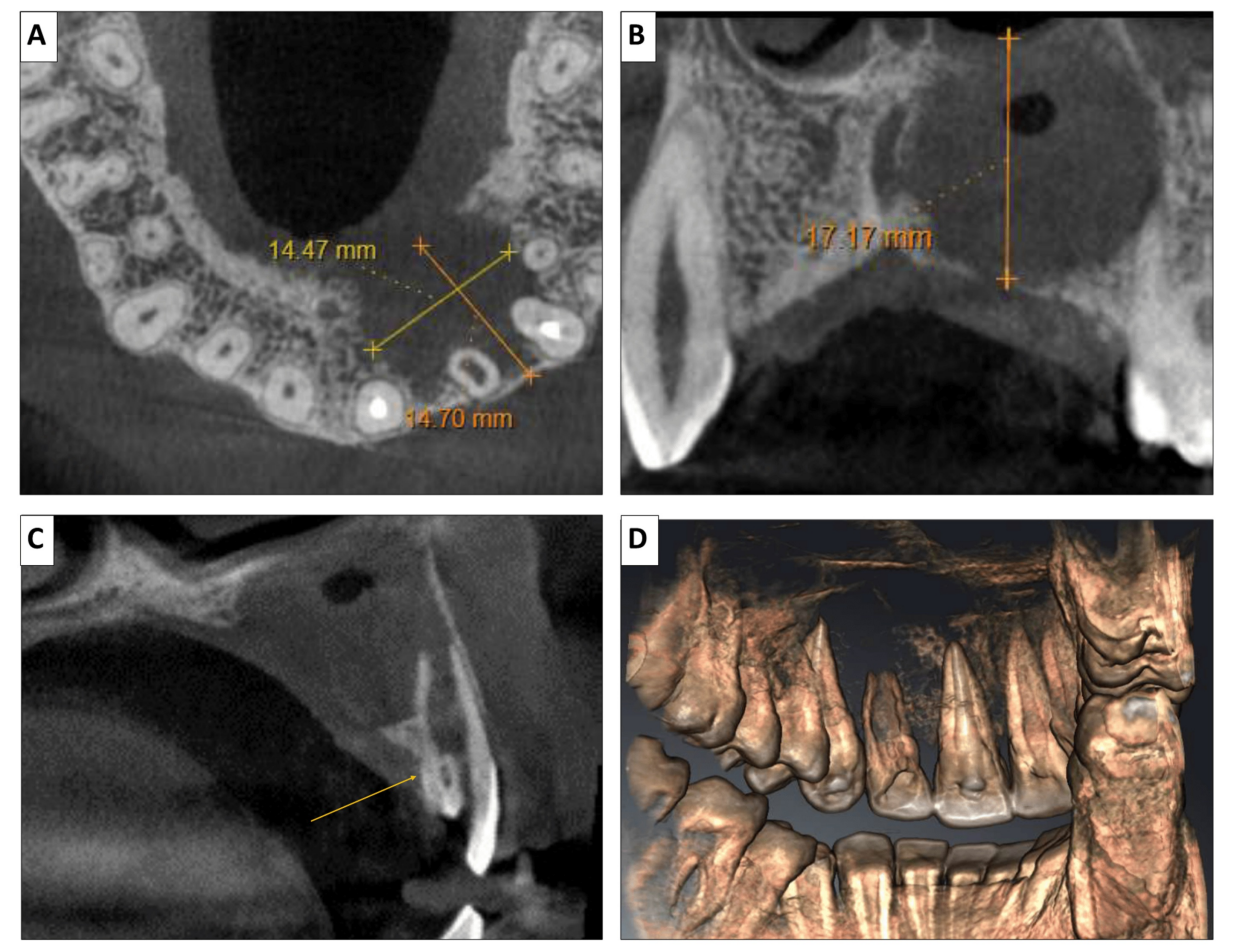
CBCT scans showing well-defined radiolucent lesion with cortication involving the maxillary left central incisor to maxillary first premolar region in (A) axial view, (B) coronal view, (C) sagital view, and (D) 3D reconstruction CBCT: cone-beam computed tomography
